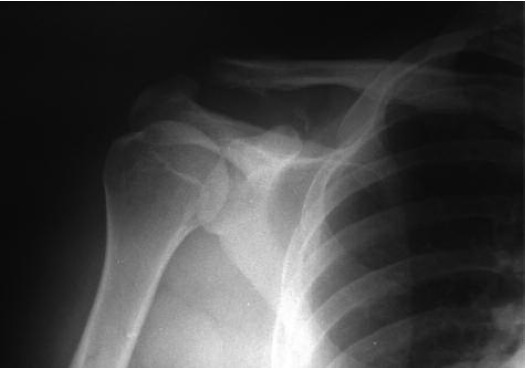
Radiograph taken after arthroscopic
